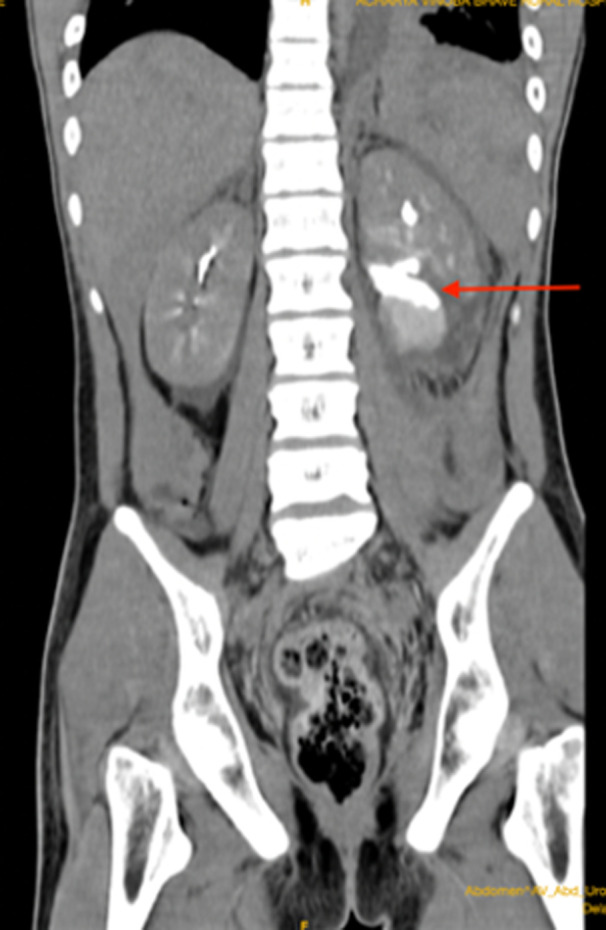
follow-up computed tomography scan showing contrast leak suggestive of urinoma (arrow)
